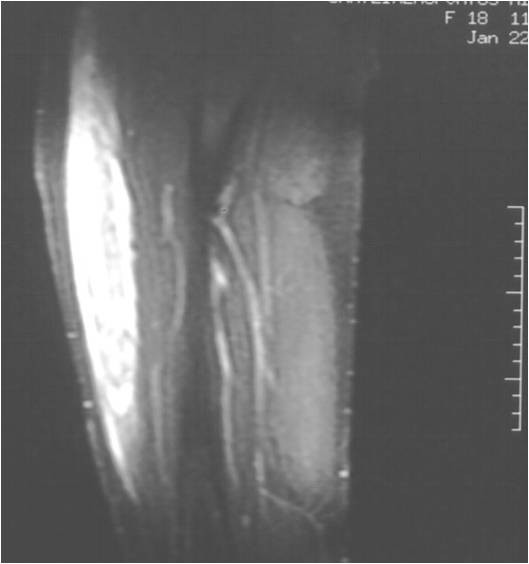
Coronal MRI section imaging haematoma of the right thigh.
